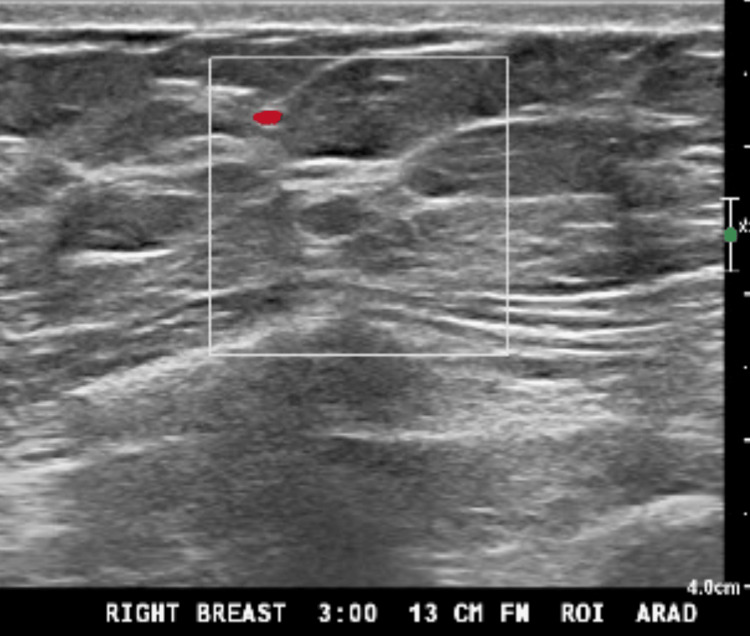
Ultrasound of the right breast, 13 cm from the nipple, with 0.4 cm mass
